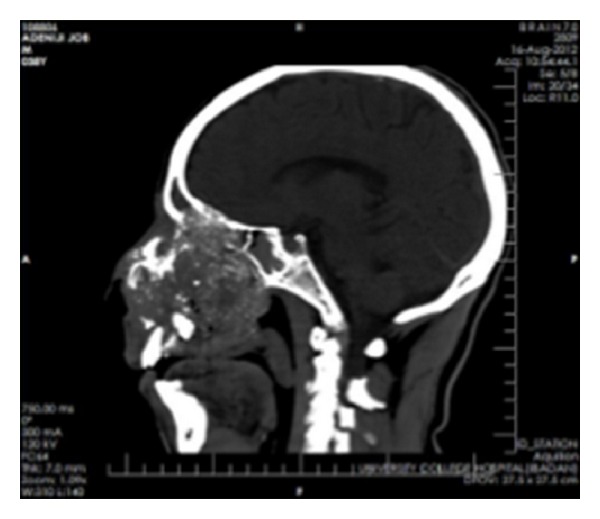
Sagittal CT showing involvement of the roof of the base of the skull.
